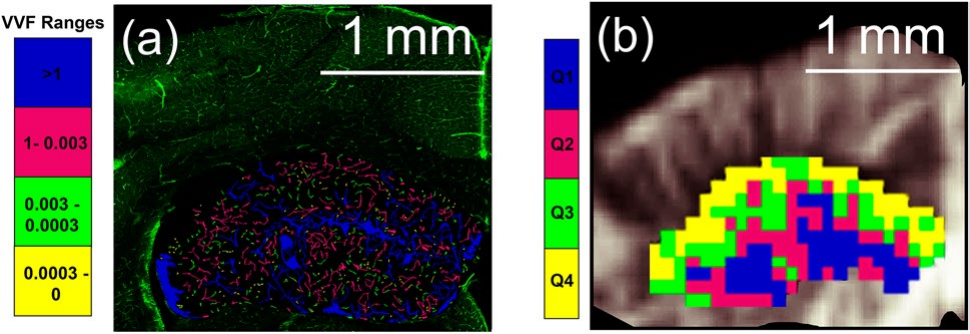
CBV and vascular structure. (a) A tile-scan image of the hippocampal endothelial vascular IB4 signal obtained by SLCM (green image) is overlapped with the 3D counter object image showing the identified ranges of volume distribution. Vessels in the 0–0.0003, 0.0003–0.003, 0.003–1 and > 1 range were coloured yellow, green, fuchsia and blue, respectively. (b) $$\mu$$Doppler image overlaid with segmented hippocampus with CBV quartile distribution. Blue, fuchsia, green and yellow corresponds to quartiles Q1, Q2, Q3 and Q4, respectively.
